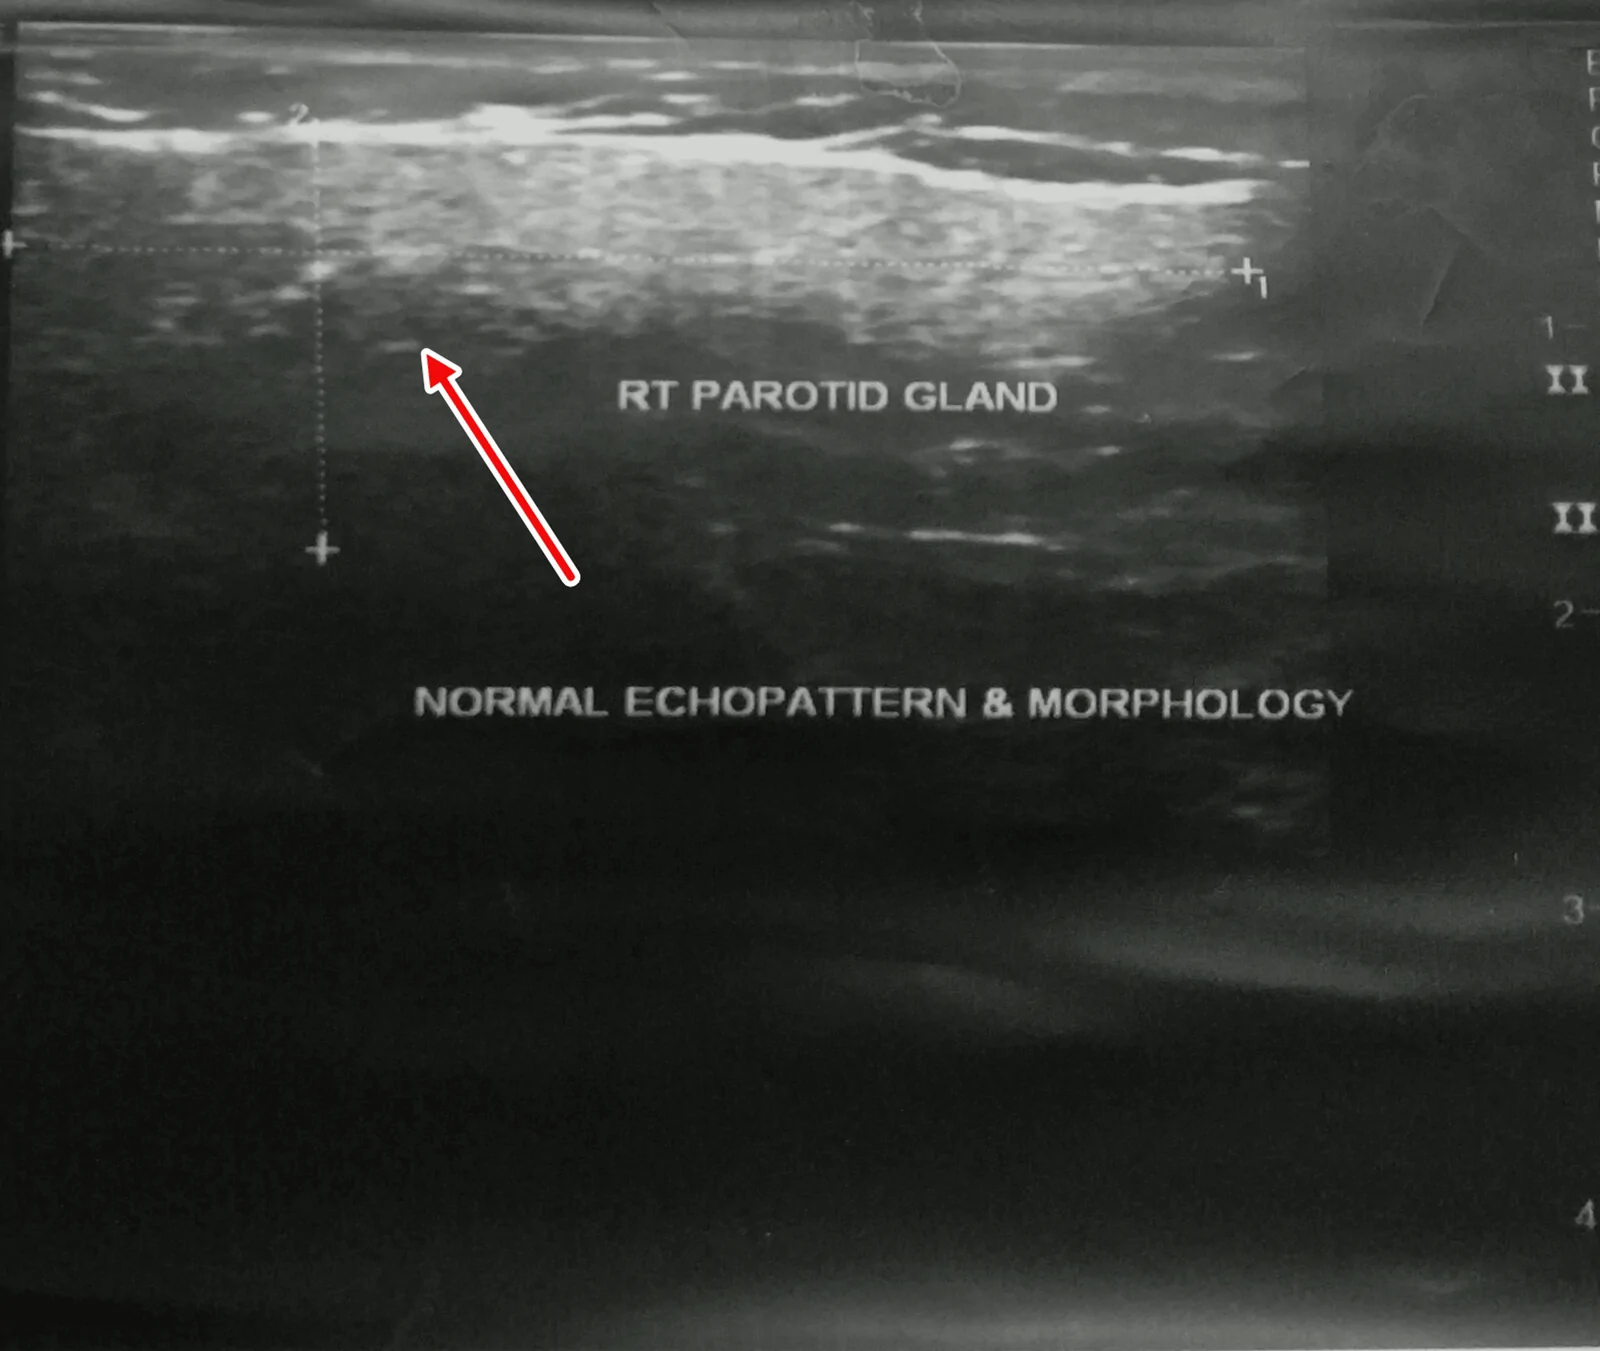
High-resolution ultrasonography showing a multilobulated mass superior to the right parotid gland
